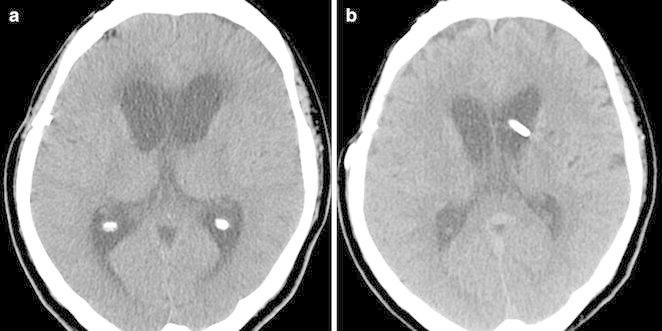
A follow-up CT scan showed a communicating hydrocephalus (a) and a ventriculo-peritoneal shunt were placed 3 months after surgery (b)
